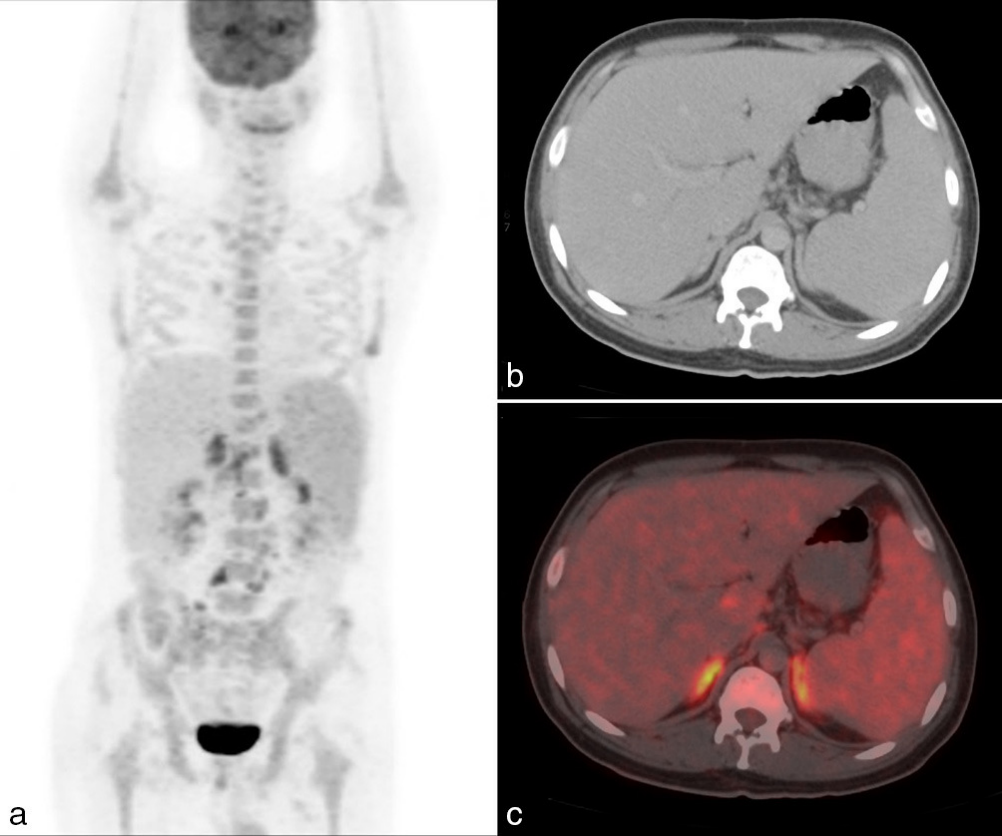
Maximal intensity projection image (a) showing intense fludeoxyglucose uptake in the bilateral suprarenal regions. Transaxial CT (b) and fused positron emission tomography/CT (c) images showing intense fludeoxyglucose uptake in the bilateral bulky adrenal glands.
